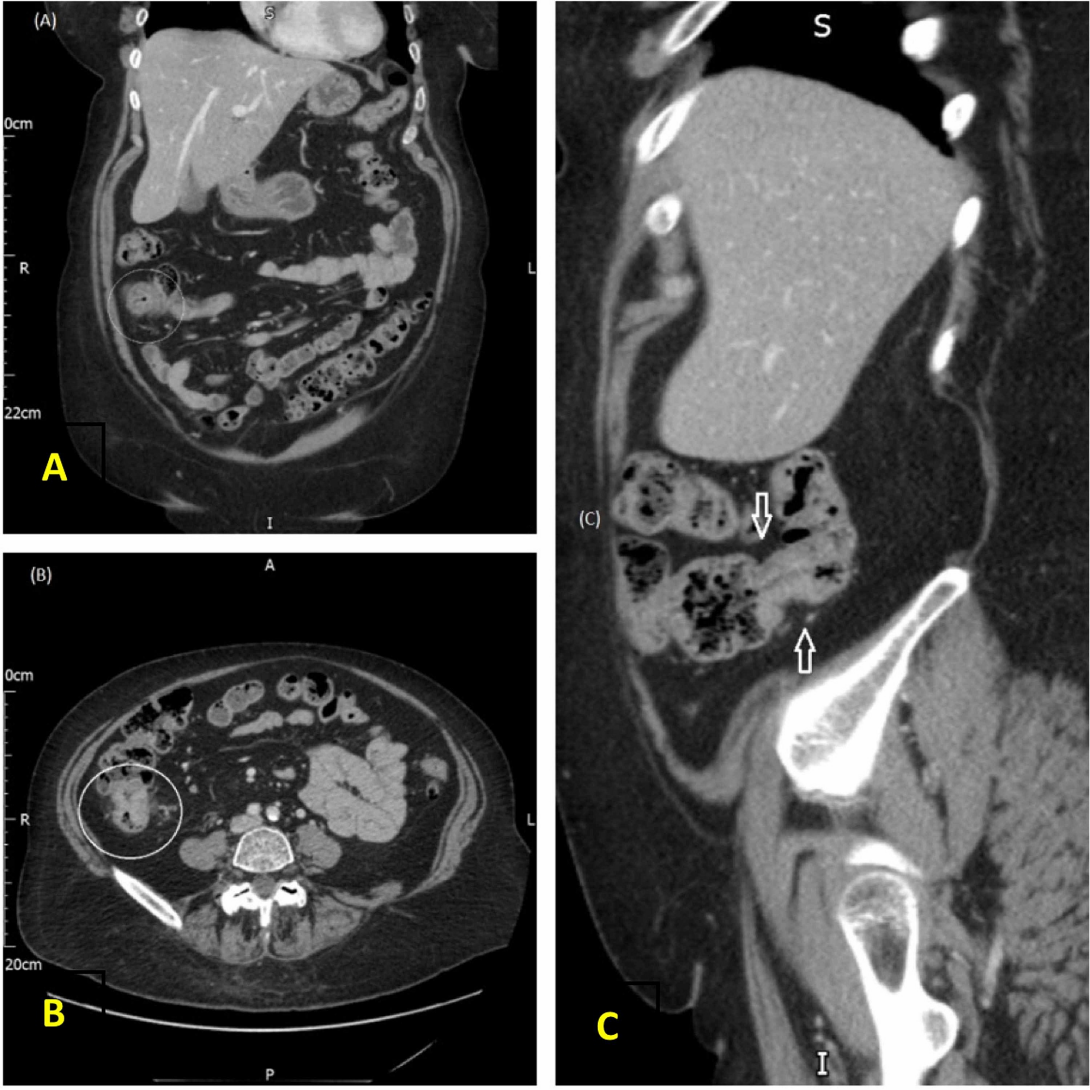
CT abdomen (A) coronal plane, (B) axial plane, (C) sagittal plane, there is a focal spot of wall thickening in the mid ascending colon.
